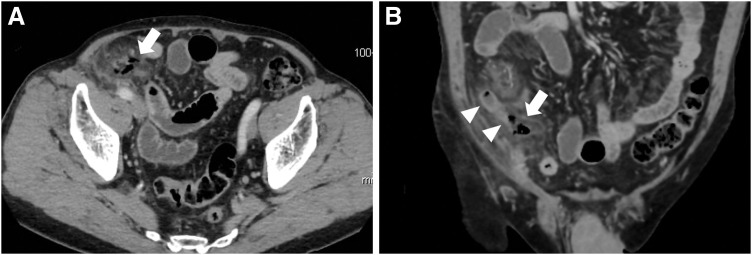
Initial CT findings. (A, B) Contrast-enhanced CT at initial presentation showing perforation of the appendix at the mid-portion with adjacent free air (arrow). Marked periappendiceal fat stranding is visible, though the contour of the appendix remains largely delineable (arrowheads).
